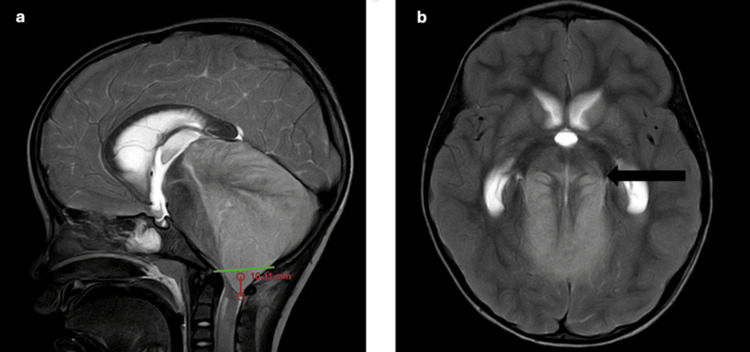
MRI brain T2-weighted (a) sagittal image showing the descent of the cerebellar tonsil by 10 mm from the McRae line and (b) axial image showing upward transtentorial herniation with obliteration of quadrigeminal cisterns and compression of the posterior aspect of midbrain giving “spinning top” appearance MRI: magnetic resonance imaging
